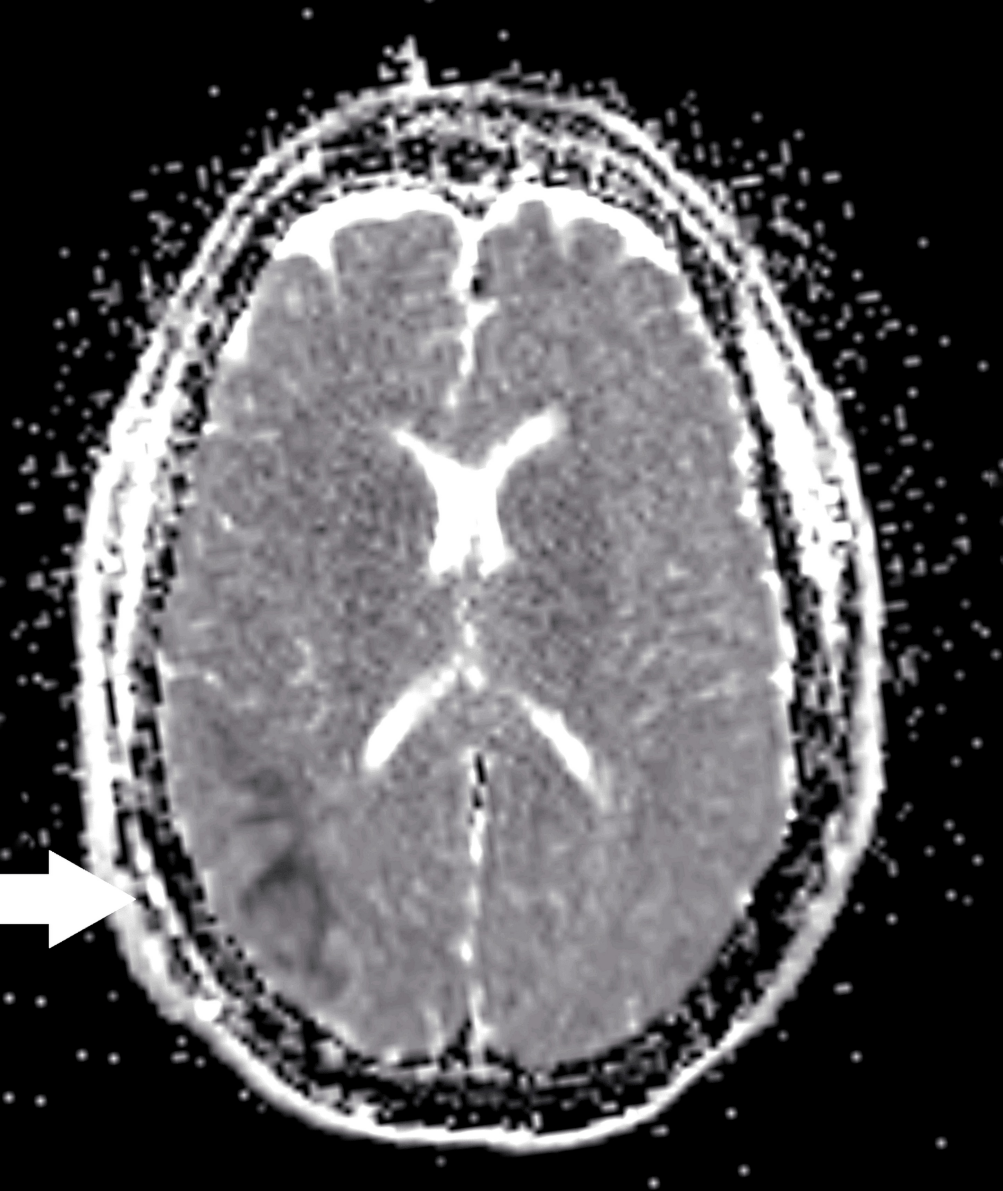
Axial ADC map of the brain MRI Axial ADC map corresponding to the DWI sequence, demonstrating reduced signal intensity in the right parietal cortex and adjacent subcortical white matter (white arrow). This finding correlates with the area of restricted diffusion seen on DWI, consistent with acute cortical involvement. ADC: apparent diffusion coefficient; MRI: magnetic resonance imaging; DWI: diffusion-weighted imaging
